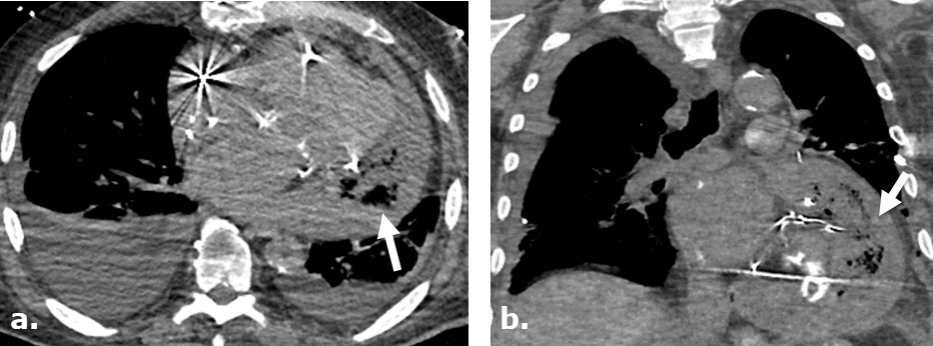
Surgicel mimicking infected pericardial fluid following cardiothoracic surgery complicated by sepsis and shock. Axial (a) and coronal (b) non-contrast-enhanced CT images of a 75-year-old male following pericardial washout and irrigation of an infected pericardial effusion demonstrates large complex gas-containing pericardial effusion, mimicking infected collection. Surgical note indicates intraoperative utilization of Surgicel for hemostasis.
